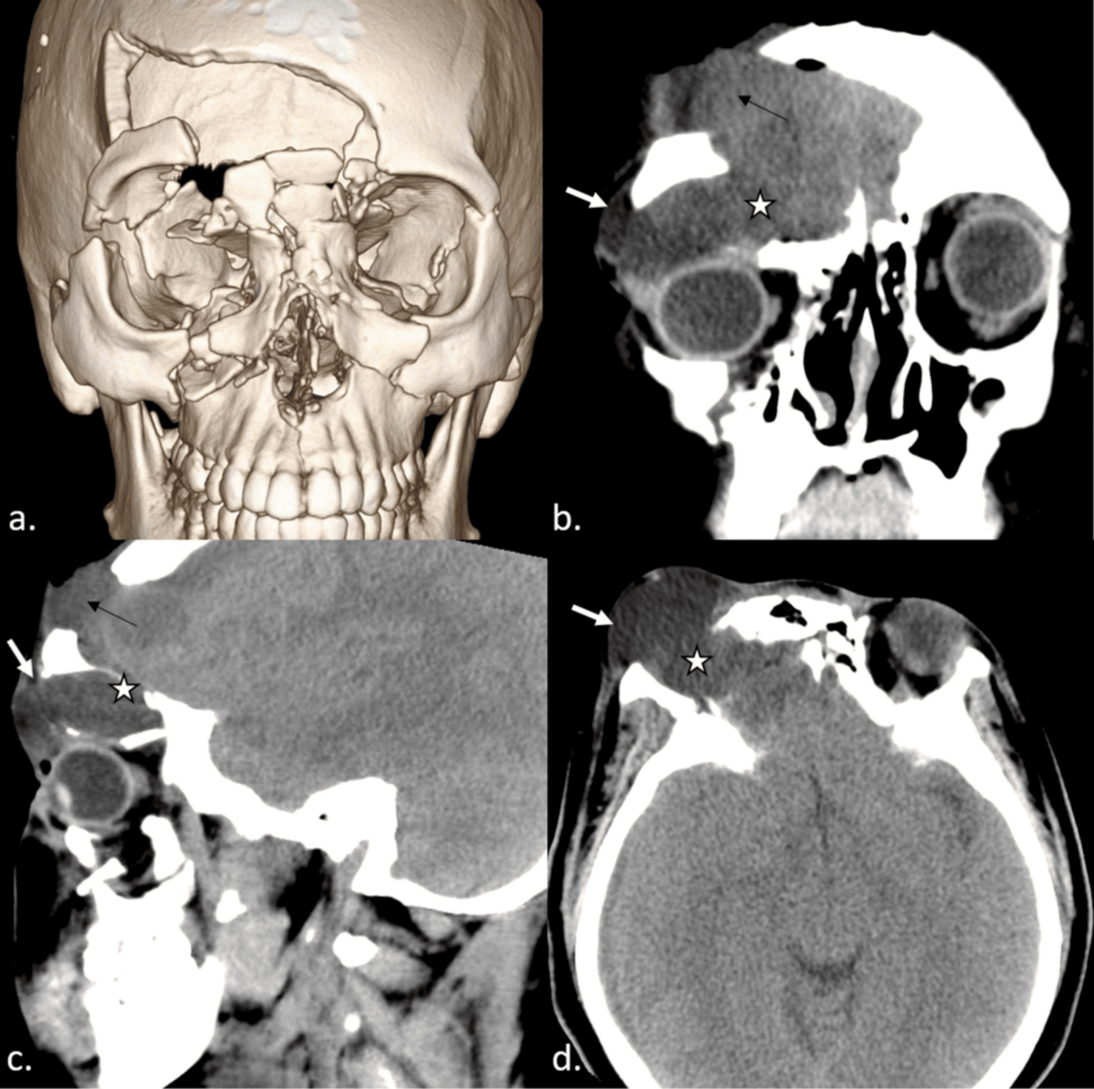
Follow-up CT images during hospital admission The 3D volume-rendered image (a) demonstrates changes from interval frontal cranioplasty. Coronal, sagittal, and axial plane images (b, c, d) demonstrate the development of a large extra-axial fluid collection occupying the right superior orbital space and extending into the right superior periorbital region (white arrows in b, c, and d) where it causes conspicuous bulging of the skin surface; this fluid collection also displaces the globe inferiorly. This collection originates from the intracranial compartment through the right orbital roof fracture defect (stars in b, c, and d) consistent with a pseudomeningocele. Coronal and sagittal images (b and c) also demonstrate an additional area of a pseudomeningocele (black arrows) extending through the right frontal calvarial defect into the forehead scalp. CT: computed tomography, 3D: three dimensional
